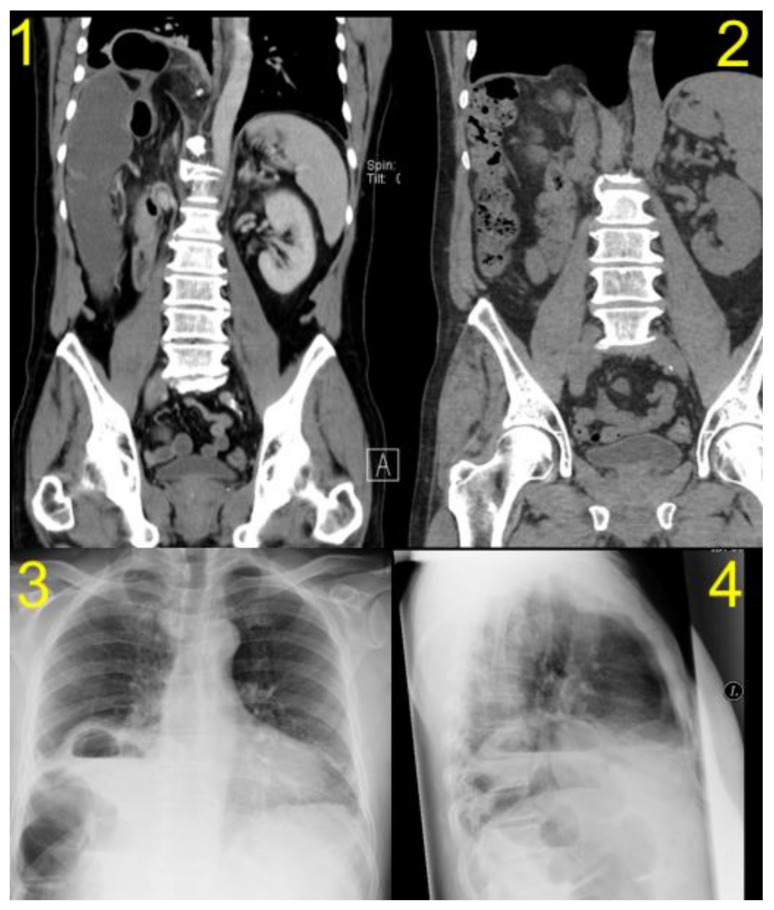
Pre- and postoperative CT scans of one of the patients with diaphragmatic hernia (DH) after right liver resection: (1) preoperative computed tomography (CT) scan, (2) follow-up CT scan showing the recurrence of DH after primary suture repair, (3) and (4) chest X-ray images before DH repair.
